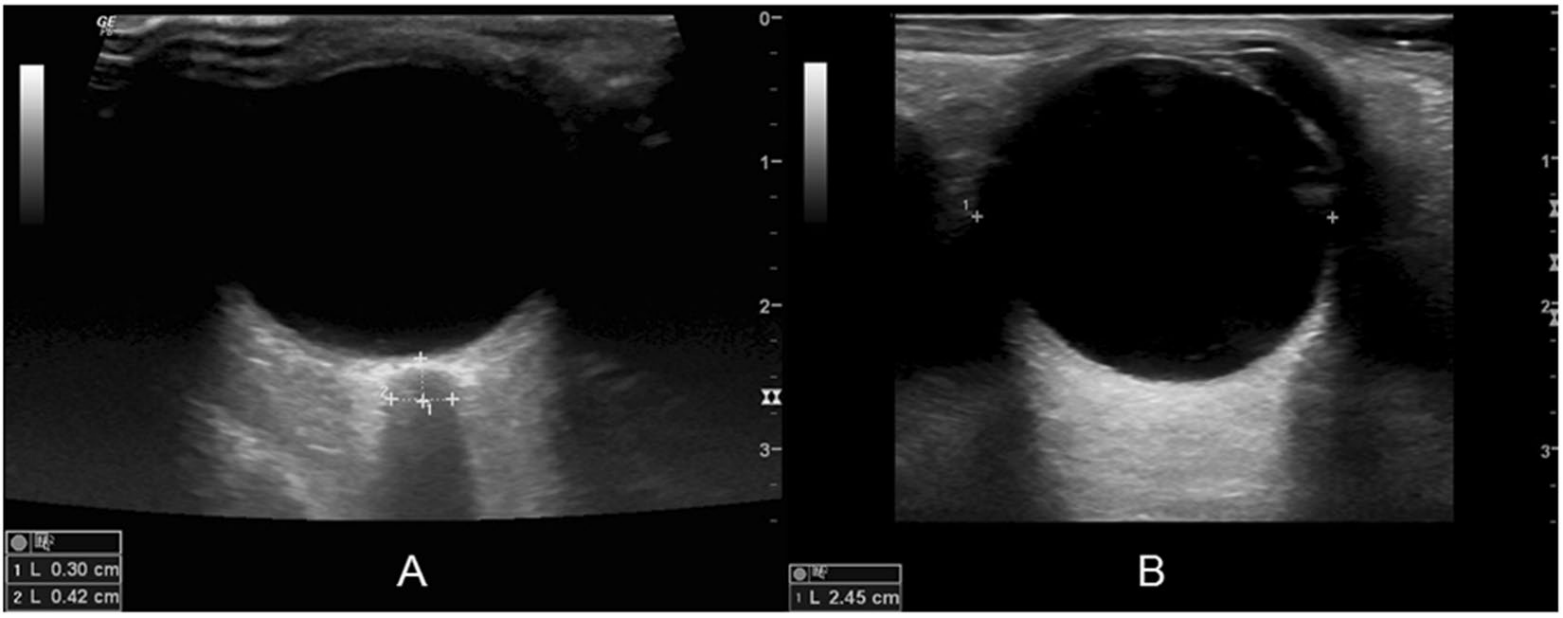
A sample ultrasonographic image for estimation of the ONSD (A) and ETD (B). (A) ONSD (measurement “2”) is measured 3 mm behind the globe using an electronic caliper along the axis (measurement “1”). *Abbreviation*s: ONSD, optic nerve sheath diameter; ETD, eyeball transverse diameter.
